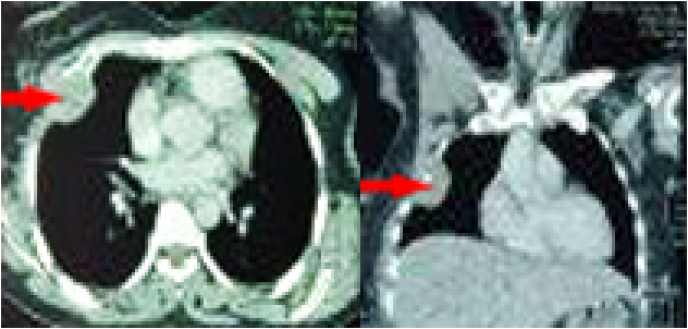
Thoracic CT scan showing a right anterior chest wall tumor.
